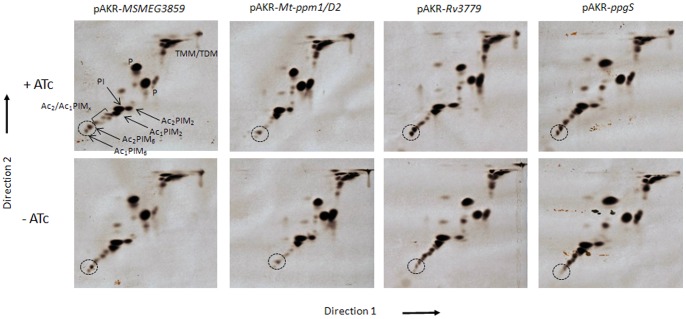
Complementation of the ΔMsPpm conditional mutant. 2D-TLC analysis of [^14^C]-labelled polar lipids from the ΔMsPpm conditional mutant complemented with (A) pAKR- *MSMEG3859* (B) pAKR-*Mt-ppm1/D2* (C) pAKR-*Rv3779* (D) pAKR-*ppgS*. Equal counts of polar lipid extracts (50 000 cpm) were applied to Silica Gel 60 F_254_ (Merck 5554) aluminium-backed TLC plates and developed using solvent system E for polar lipids: CHCl_3_/CH_3_OH/H_2_O (60∶30∶6, v/v/v) in the first direction and CHCl_3_:CH_3_OOH: CH_3_OH:H_2_O (40∶25∶3∶6, v/v/v/v) in the second direction. Polar lipids were visualized by 48 h exposure on x-ray films by autoradiography (Kodak Biomax MR film). PIM intermediates are shown by arrows (x = 3–5) and the dotted circle indicates the position of the higher PIMs on the TLC plates.
